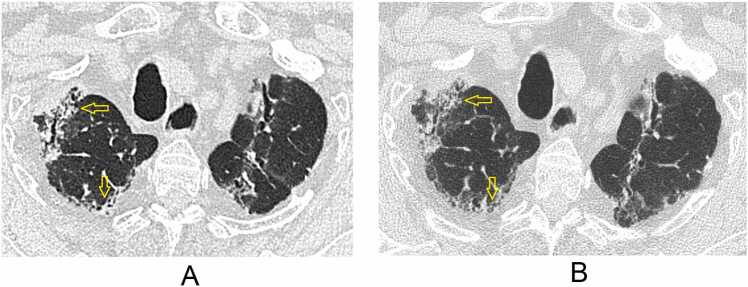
Example of VGA +1 for reticulations in IQ40*. Patient with RAS received a score of +1 for reticulations by multiple readers, suggesting improved visibility without perceived diagnostic impact. (A) UHR-PCCT shows sharp delineation of fine reticulations in the right upper lobe (arrows); (B) HR-EIDCT shows more unsharp/hazy appearance of the same area of fine reticulations (arrows), although the diagnosis of reticulations and RAS could still be made. Abbreviations: RAS, restrictive allograft syndrome; UHR-PCCT, ultra-high-resolution photon-counting CT; HR-EIDCT, high-resolution energy-integrating-detector CT. The most experienced reader was R4 with 35 years’ experience as a thoracic Radiologist. R2 has 8 years and R3 has 2 years of experience as a thoracic Radiologist. R1 is a radiology resident in training to become a thoracic Radiologist. * Green: most frequent score.
